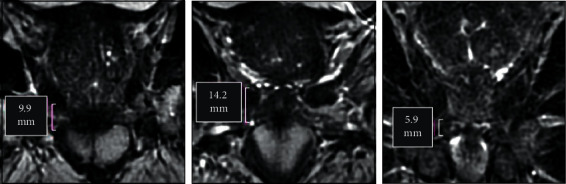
Anatomic variation at the prostate apex. Length of the urogenital diaphragm measured on MRI in the coronal plane is shown.
